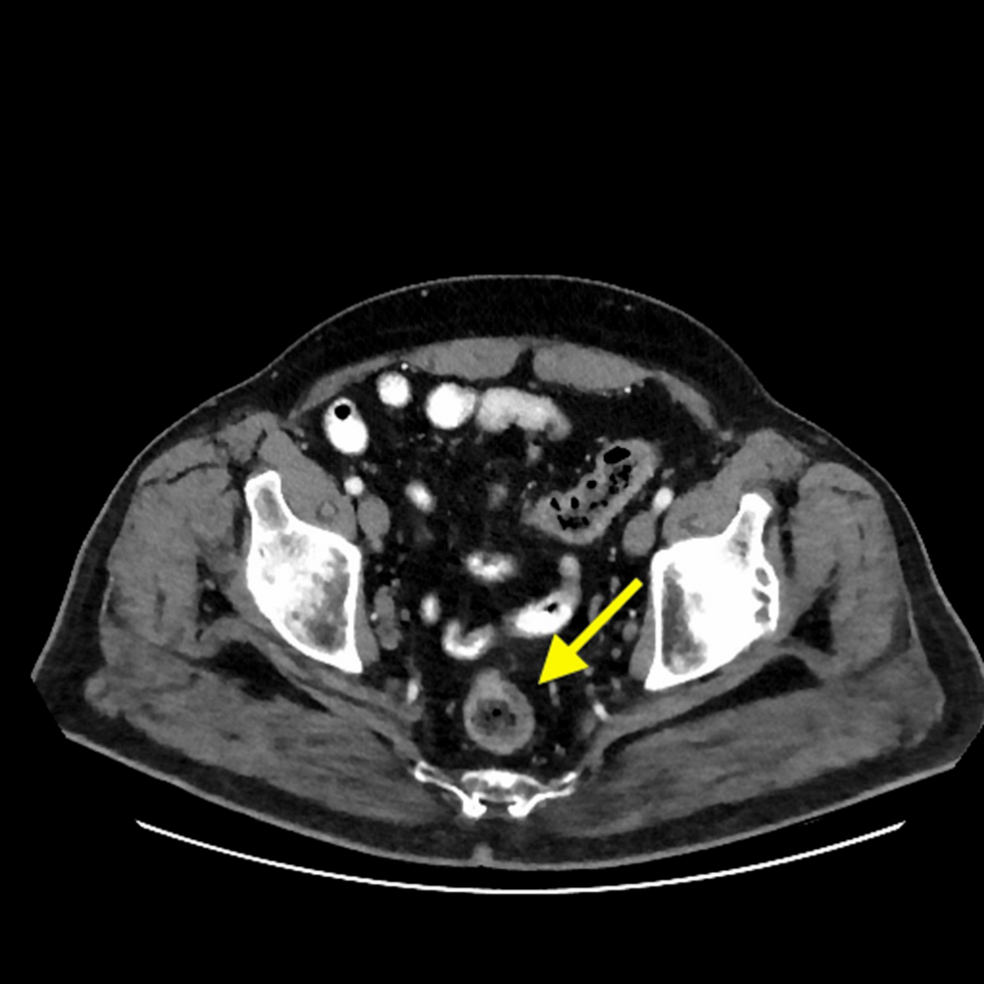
Cross-sectional image of the computed tomography (CT) of the abdomen showing rectal wall thickening
